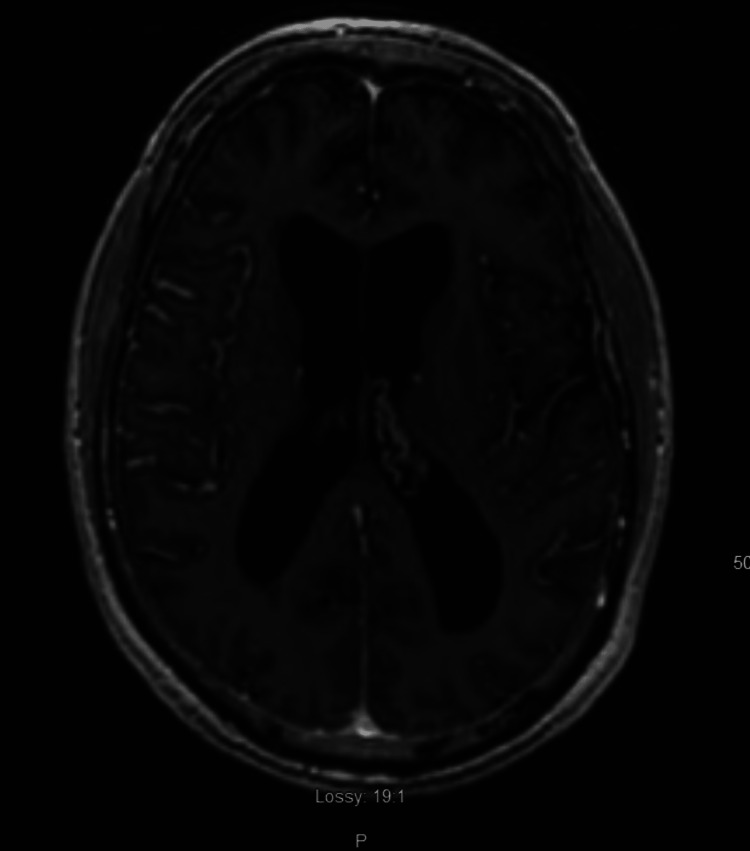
Axial MRI images demonstrating leptomeningeal enhancement in the insular cortex and frontal cortex
